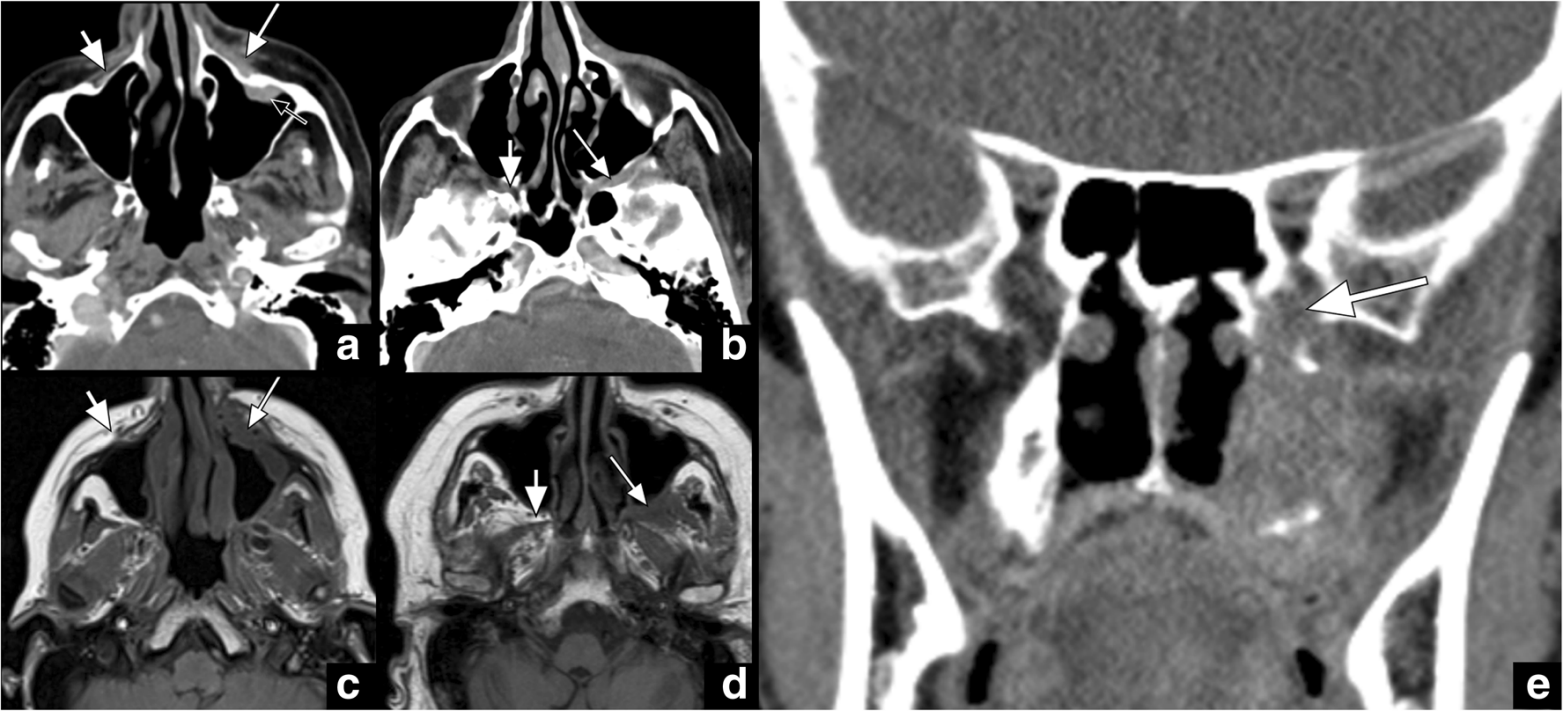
Axial CT (a and b) and axial T1-weighted (c and d) images show obliteration of the preantral fat pad on the left (*long white arrow* in a and c) when compared to its normal appearance on the right (*short white arrow* in a and c). In addition, note the enhancing soft tissue within the maxillary sinus (*black arrow* in a) as well as the obliteration of the fat pad in the left pterygopalatine fossa (*long white arrow* in b and d) compared to its normal appearance on the right (*short white arrow* in b and d). The obliteration of the fat pad in the left pterygopalatine fossa (*long white arrow*) can also be well appreciated on coronal images (e). These signs are indicative of PTNS along V2 in this patient with history of left cheek skin cancer presenting with V2 numbness
